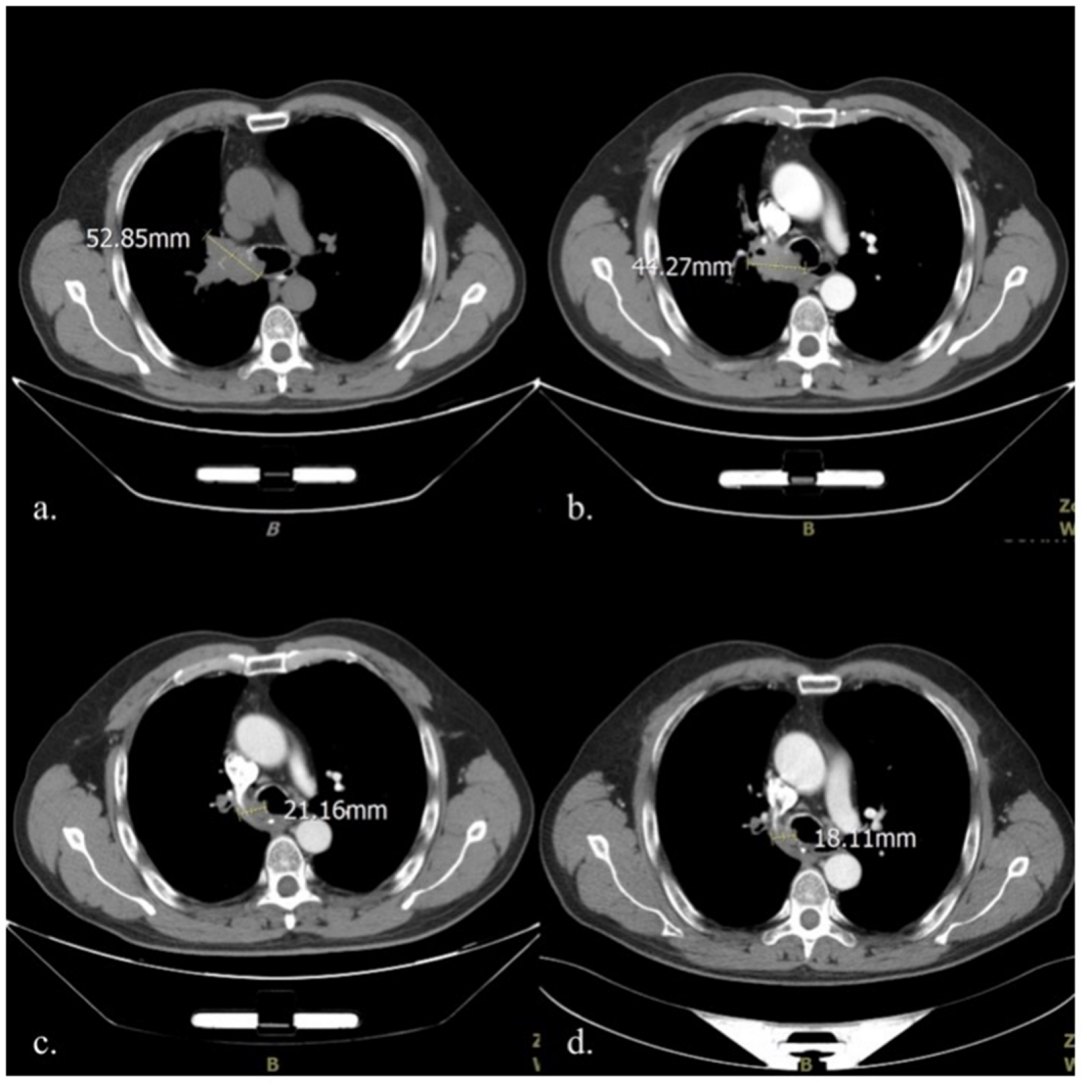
Comparison of mediastinal window CT images in four different periods: (A) Before treatment initiation (2020-10-12); (B). After two cycles of chemotherapy (2020-11-25); (C) At the end of the 5^th^ fractionation of radiotherapy (2020-12-9); (D) At the end of the 10^th^ fractionation of radiotherapy (2020-12-16). CT, computed tomography.
